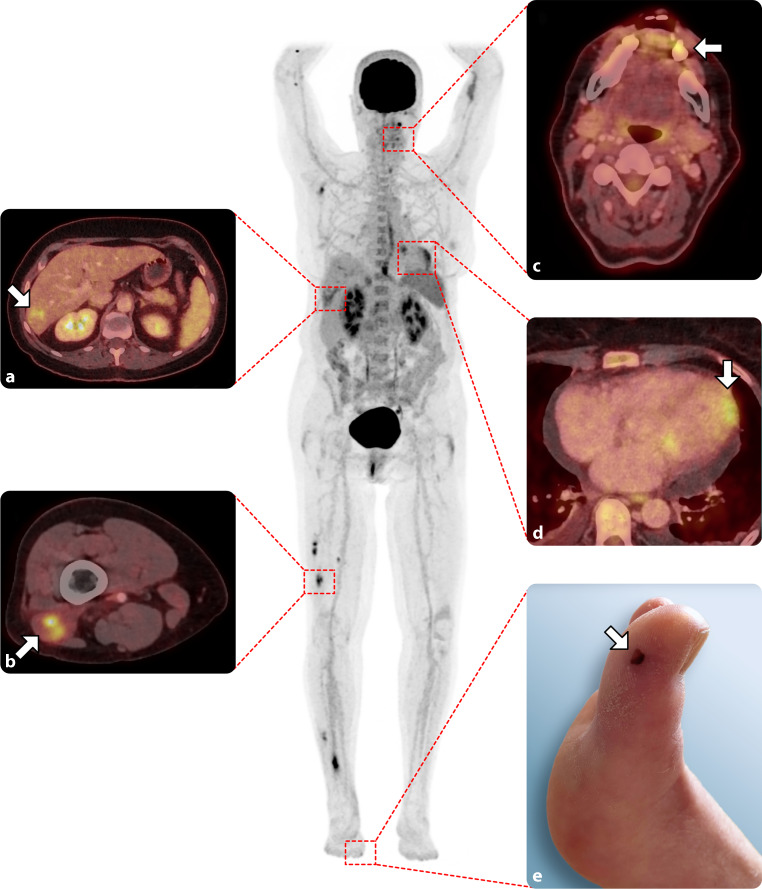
Total body maximum intensity projection with slices of fused ^18^F‑fluorodeoxyglucose positron emission tomography/computed tomography images showing embolisation to a right liver lobe, b biceps femoris muscle, c left mandibula (with possible primary (dental) focus) and d left ventricular apex. e Discrete focal FDG uptake is present in Jane Way lesion on medial side of right big toe
